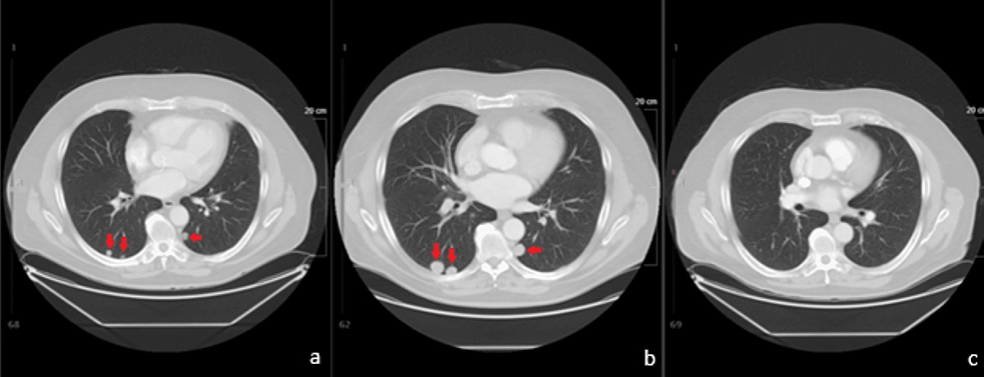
Contrast-enhanced computed tomography scan: (a) before treatment showing multiple pulmonary nodules (red arrows), (b) pulmonary lesions increased at six months following treatment, and (c) the lesions decreased and disappeared completely at 14 months
